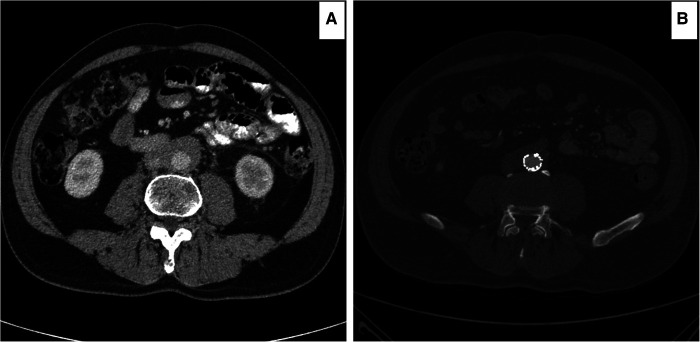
Computed tomography angiography (CTA) images showing improvement of the peri-aortic soft tissue enhancements following endovascular aneurysm repair (EVAR). (A) Post 16 months on optimal medical therapy, the peri-aortic soft tissue enhancement of 11 mm. (B) One year post-EVAR, all symptoms and signs disappeared with peri-aortic soft tissue enhancement of 3mm.
